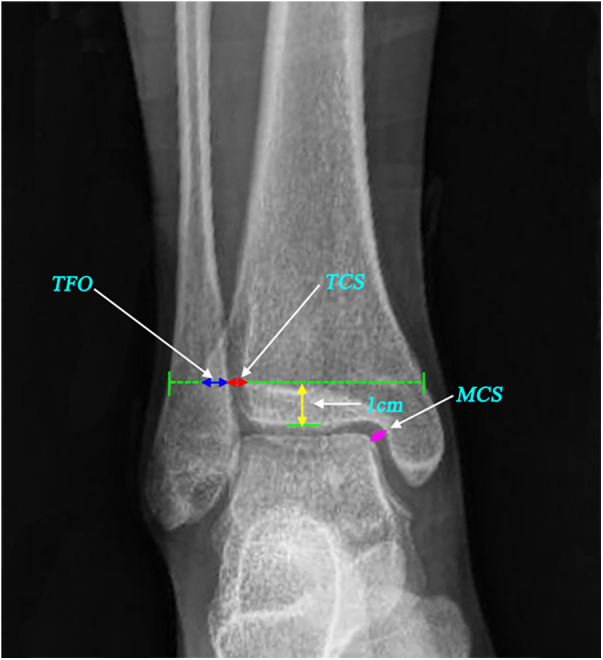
Measurements for evaluating syndesmotic reduction in the radiographs. Blue double-headed arrow: tibiofibular overlap (TFO); red double-headed arrow: tibiofibular clear space (TCS); pink double-headed arrow: medial clear space (MCS).
